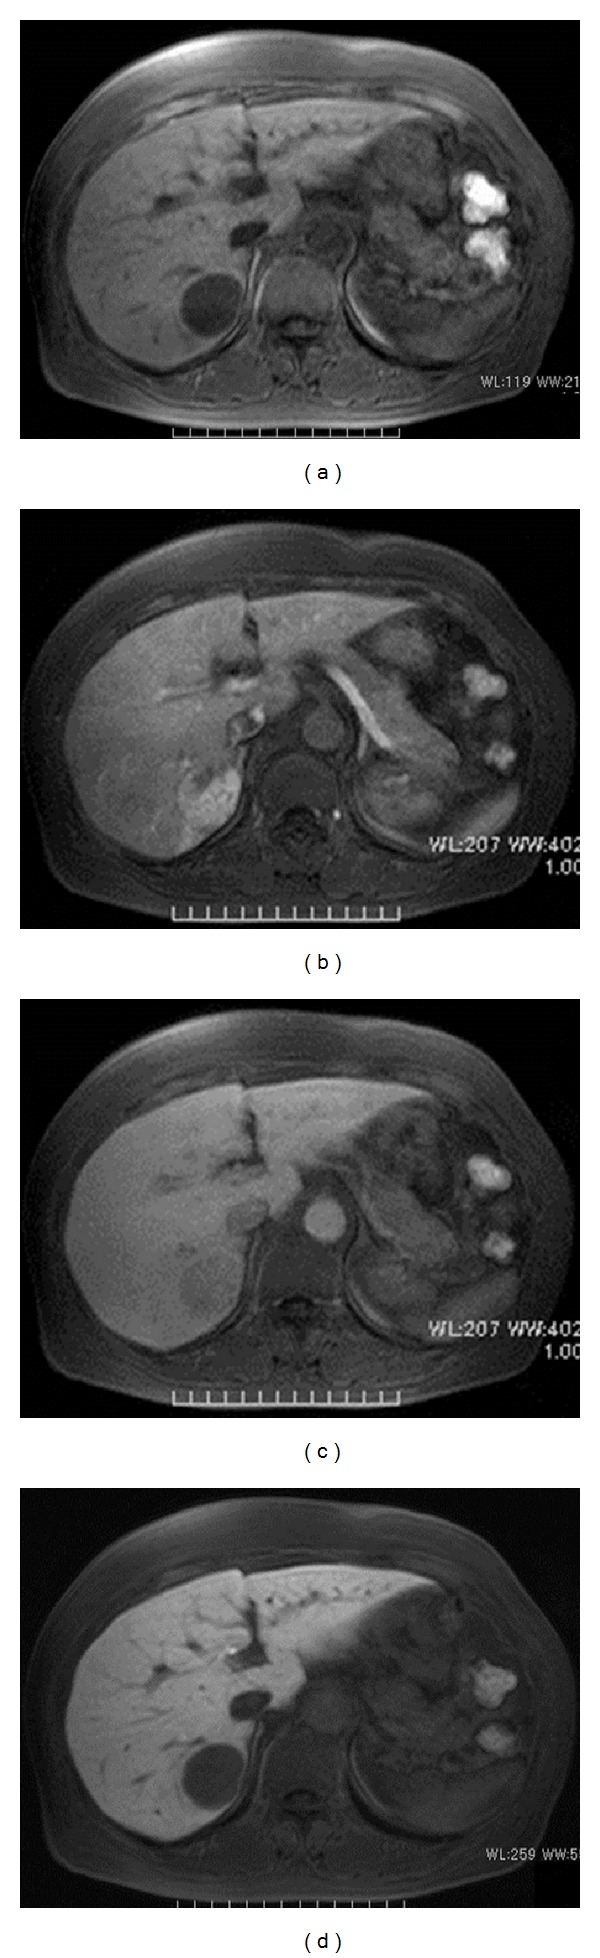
Dynamic contrast enhancement MRI with a hepatocyte-specific contrast agent (gadolinium-ethoxybenzyl-diethylenetriamine pentaacetic acid). Plain T1-weighted MRI revealed a low-intensity tumor (a), arterial phase hypervascularity (b), and a defective area visible in the late phase (c) and in the hepatic cellular phase (d).
